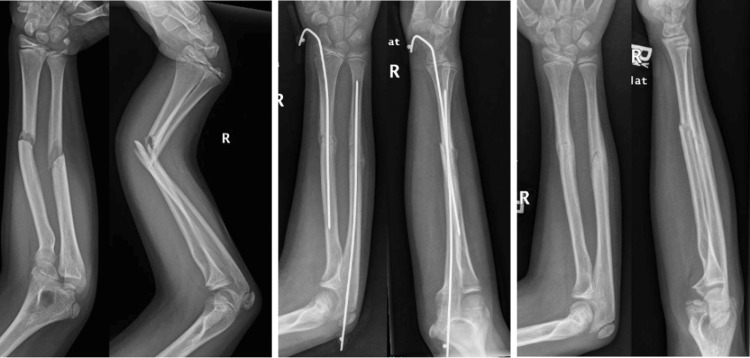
Preoperative, four-week postoperative and 10-week postoperative radiographs of an 11-year-old male treated with percutaneous intramedullary pinning of a right type 1 open both bone fracture
